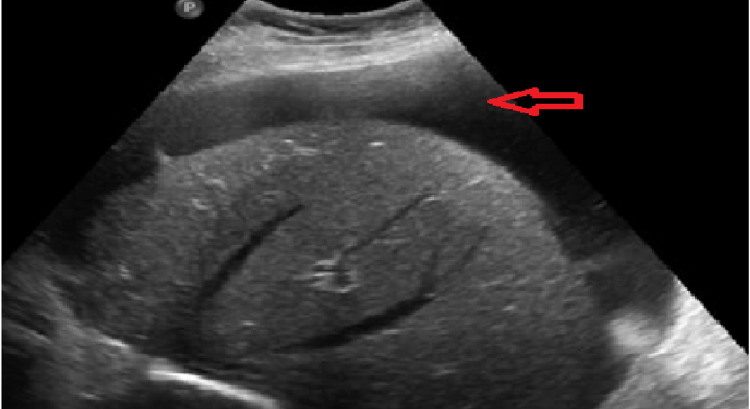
Abdominal ultrasound demonstrating ascitic fluid around the liver (arrow)
